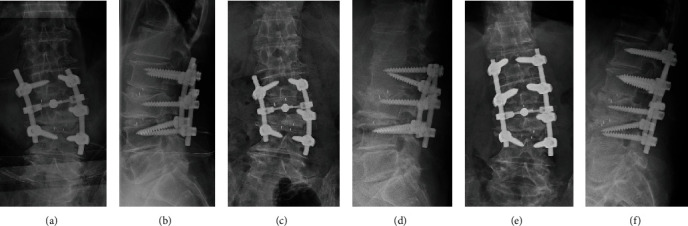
Radiographs at (a, b) immediate postoperative period, at (c, d) 4 weeks postoperatively after primary surgery, and (e, f) additional surgery.
